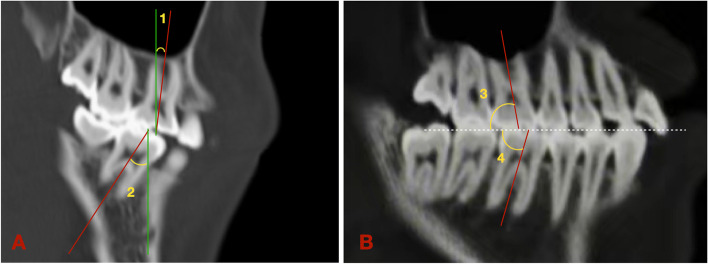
Angular measurements of right first molars on the sagittal CT image. Red line: the long axes of upper/lower first molar. Green line: planes parallel to the coronal plane. White dot line: occlusal plane. A: the angle between first molars and the coronal plane. 1: R: ∠16/Coronal; 2: R: 46/Corona. B: the angle between
